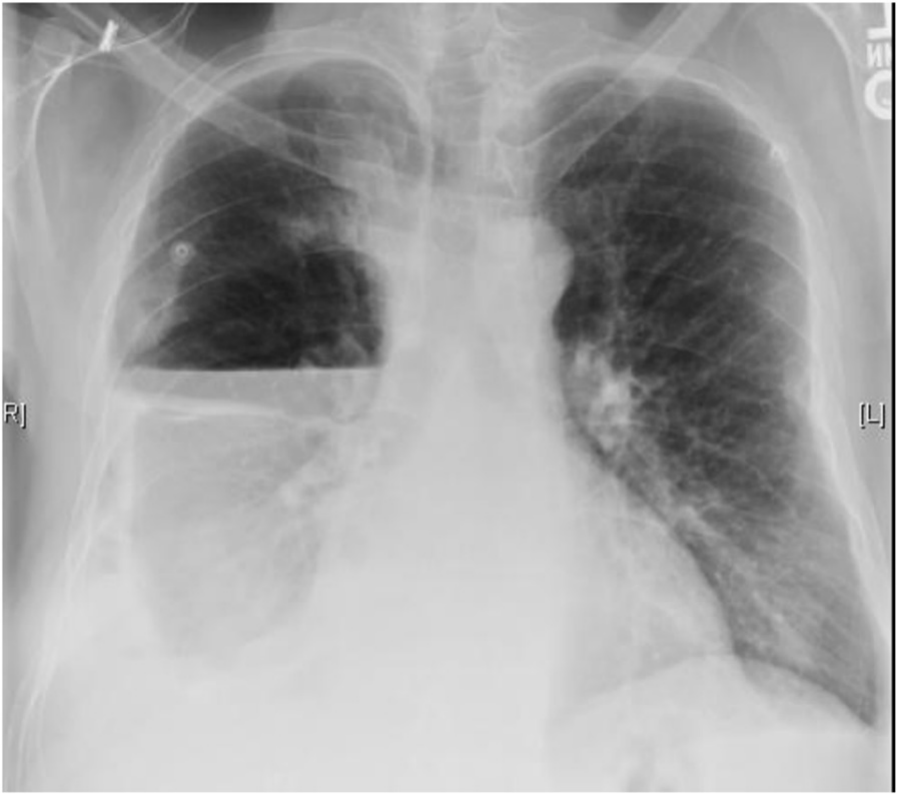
Chest x-ray after removal of indwelling pleural catheter showing a large right-sided loculated hydropneumothorax.
